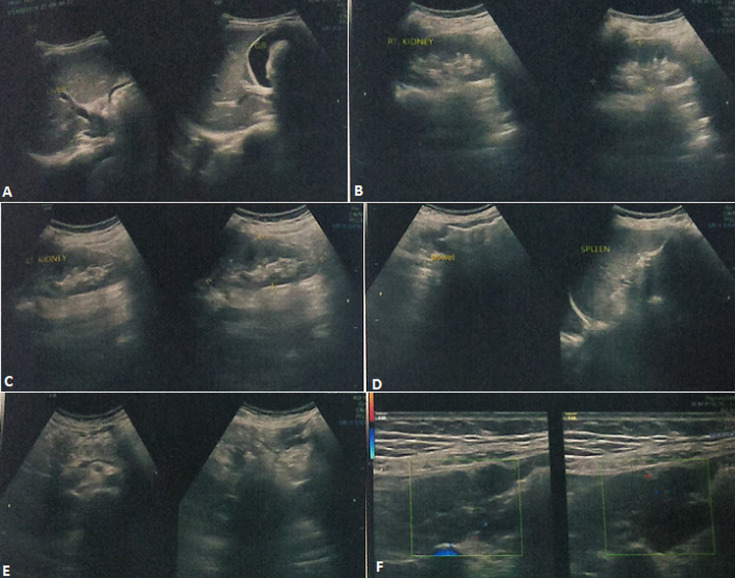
(A, B, C, D, E, F) ultrasound image taken for the first time on September 10, 2021
